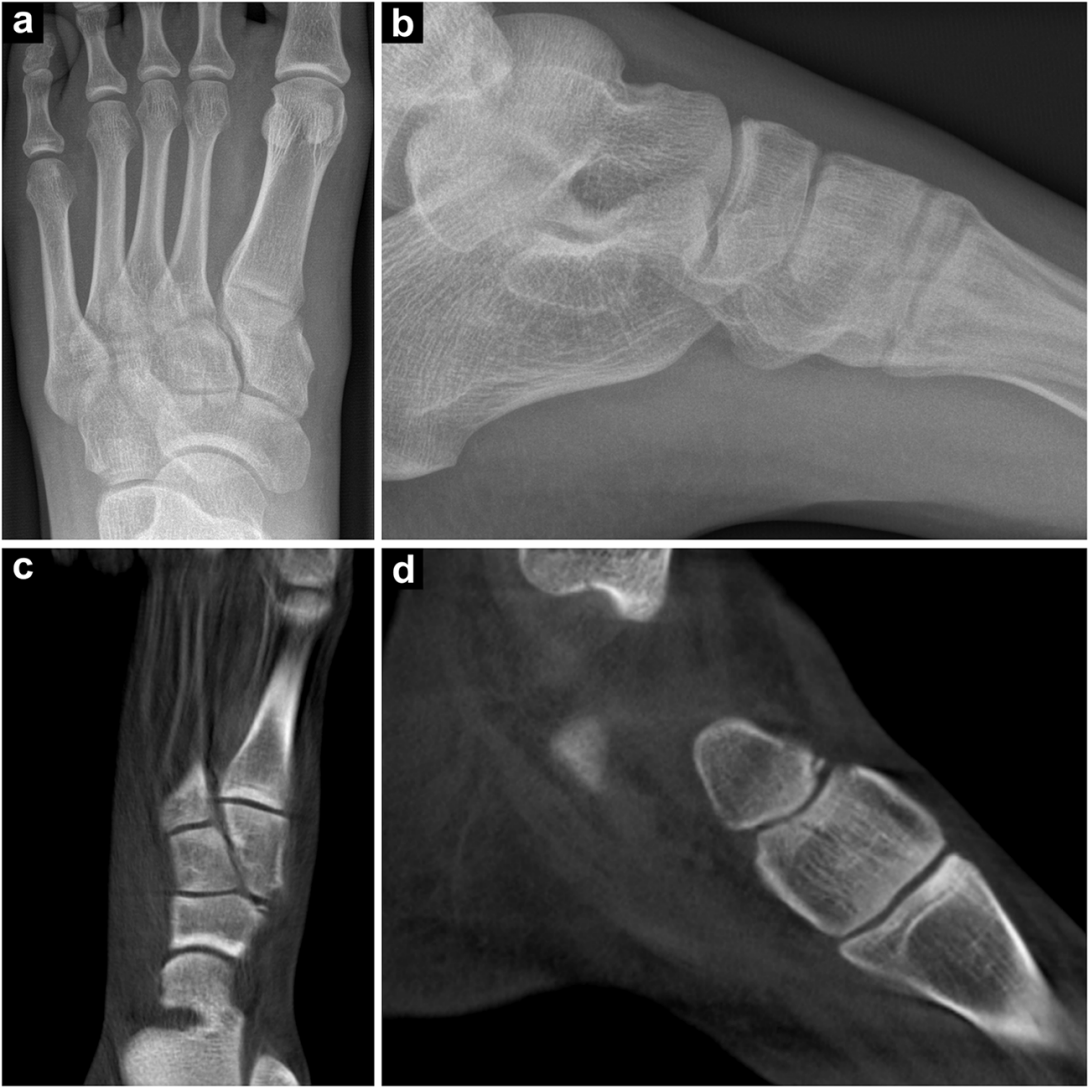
A 22-year-old male sprinter was admitted with increasing left ankle pain after an acute sprain in training. He was unable to bear weight on the left foot and stated pressure pain over the proximal first metatarsal. No fracture could be located in anteroposterior and lateral x-ray scans (a, b), whereas ensuing cone-beam CT depicted a medial avulsion fracture of the left navicular bone (c, d). Treatment was upgraded to cast immobilization
